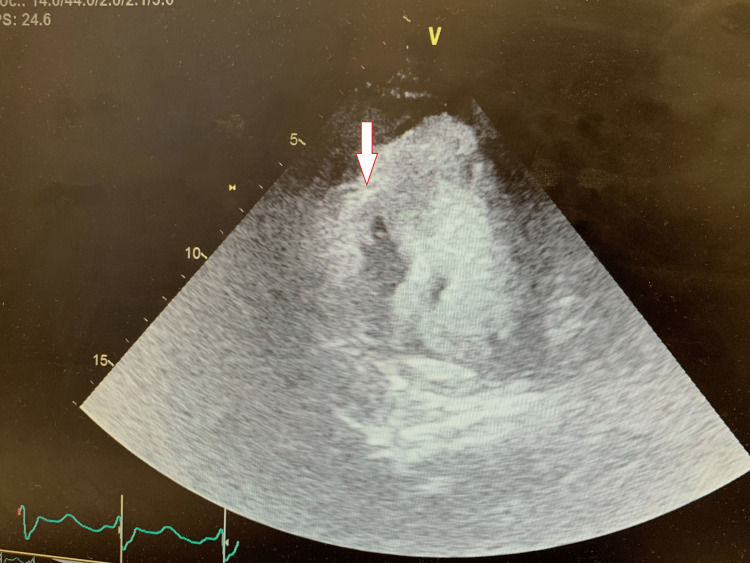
Transthoracic contrast echocardiography showing the Lumason crossing through the ventricular septal defect with a left to right shunt.
